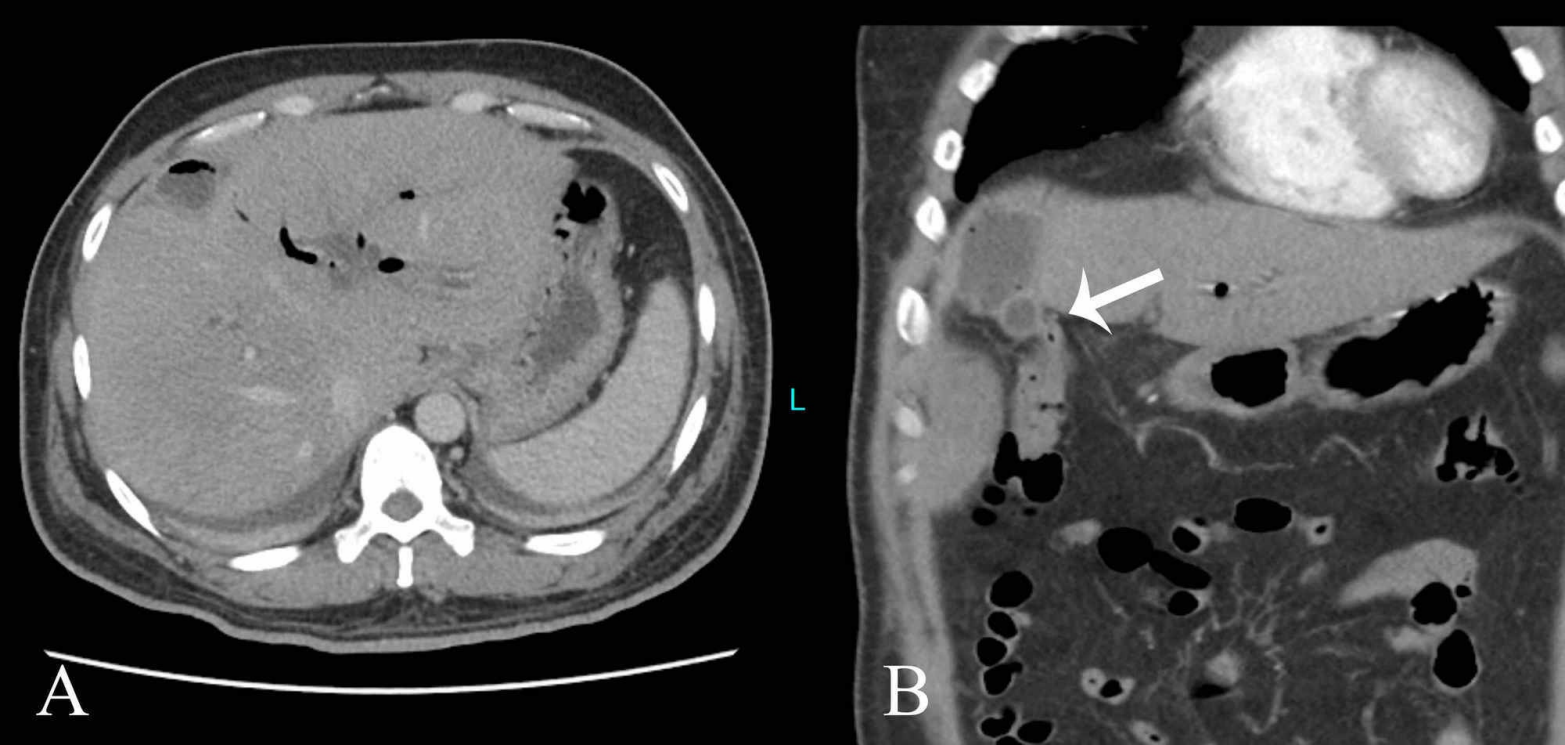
Computed tomography images A) Pneumobilia seen in the intrahepatic biliary channels and air specks noted within the gallbladder lumen. B) Fistulous communication was demonstrated between the gallbladder and colon (arrow).
